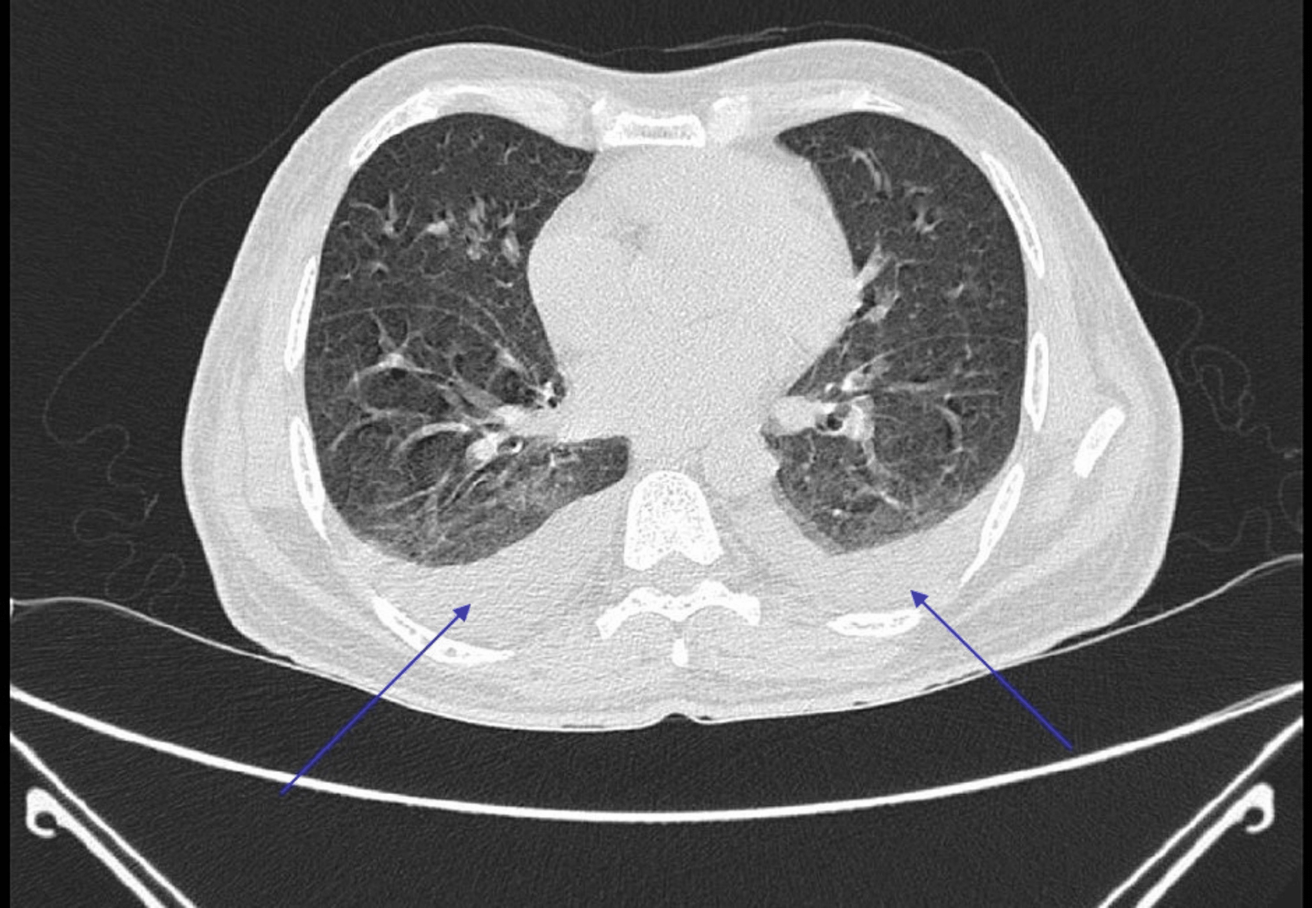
CT scan of the chest showing bilateral pleural effusion (blue arrows)
